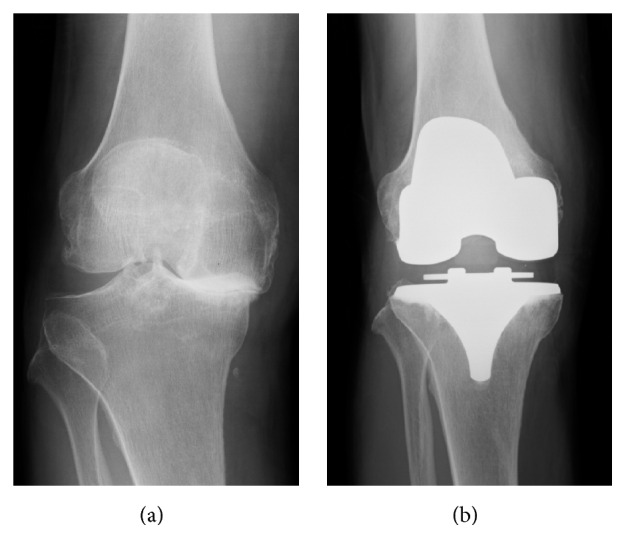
Preoperative (a) and postoperative (b) frontal radiographs of the knee.
